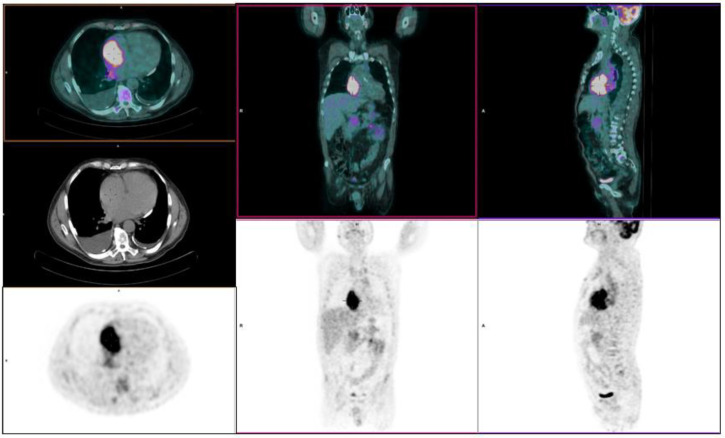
January 2020; 18F-FDG-PET/CT before therapy showing an intense uptake in the right atrium (SUV max 9.4) expression of high metabolic activity. Subsequent biopsy examination confirmed right atrial lymphoma relapse.
